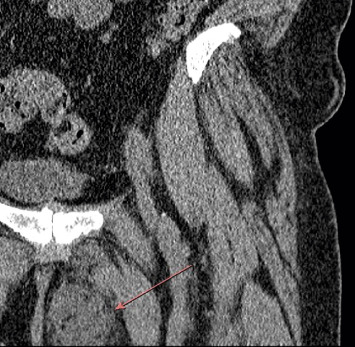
CT scan demonstrating a marginated cystic mass in the subcutaneous perineal fat.
